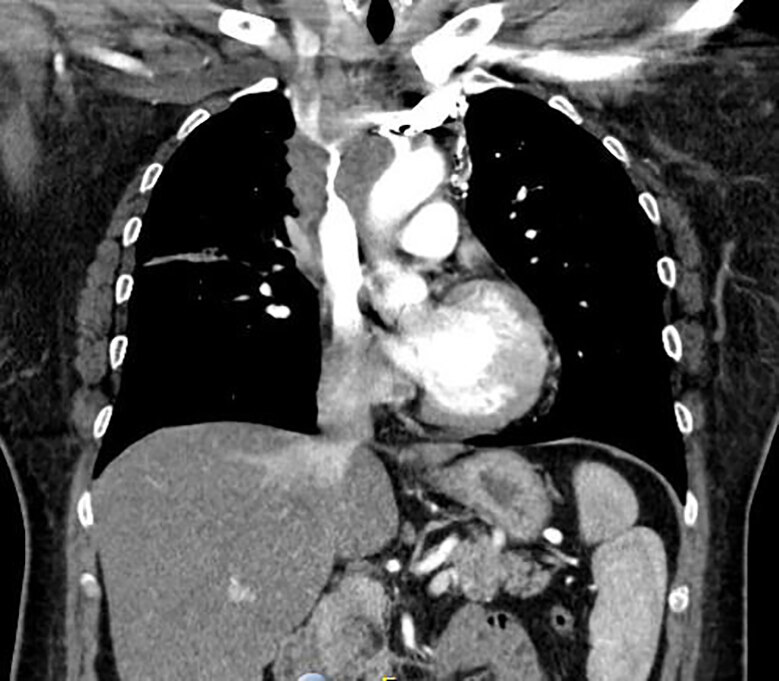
Computed tomography of the chest with intravenous contrast of a 54-year-old female with facial swelling.
